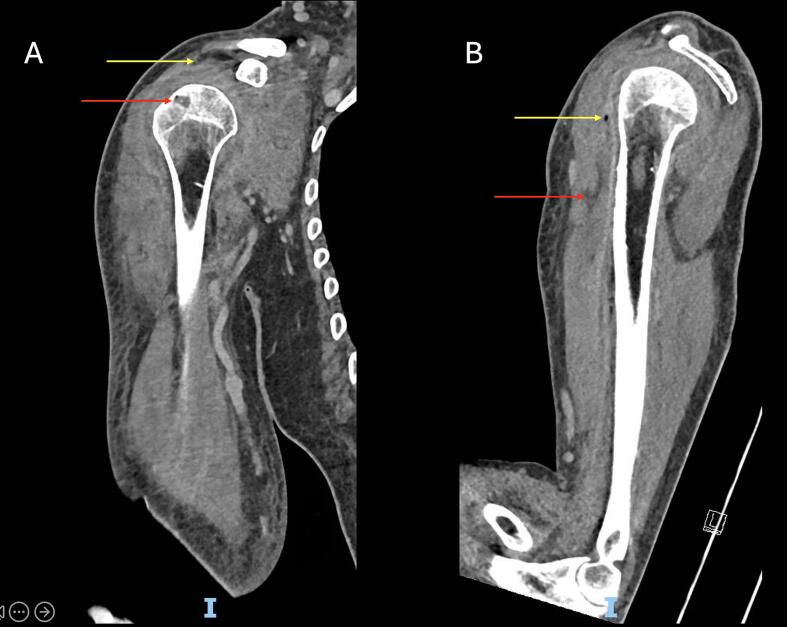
(A) Coronal CT image of Patient 2's right shoulder showing a gas-containing subacromial bursal effusion (yellow arrow) communicating with the glenohumeral joint via a full-thickness supraspinatus tear, consistent with septic arthritis. Note bone erosion and a small focus of intraosseous gas at the greater tuberosity (red arrow), consistent with osteomyelitis. (B) Sagittal CT image demonstrating a fluid collection (red arrow) with a gas locule (yellow arrow) tracking along the anterior compartment musculature of the arm, consistent with necrotising fasciitis. (For interpretation of the references to colour in this figure legend, the reader is referred to the web version of this article.)
